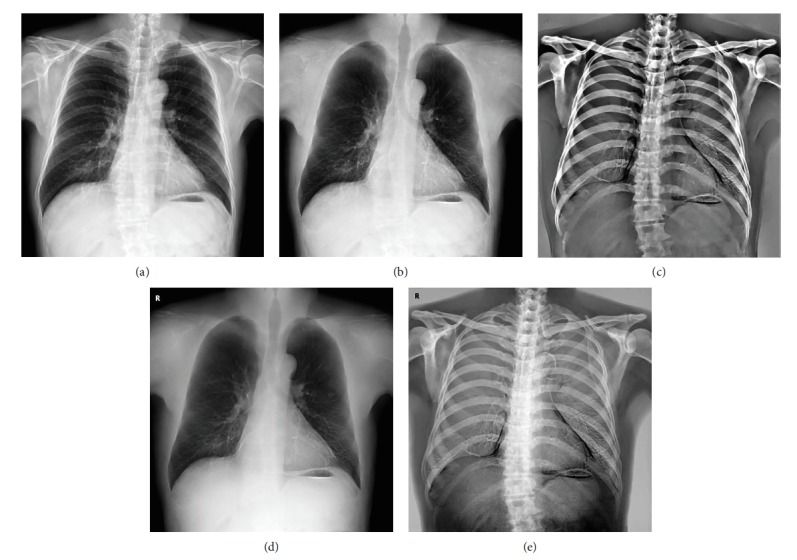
An example of the processed DES training set: (a) the standard chest radiograph; (b) the processed DES soft tissue image; (c) the processed DES bone image; (d) the soft tissue image directly obtained by the DES system; (e) the bone image directly obtained by the DES system.
